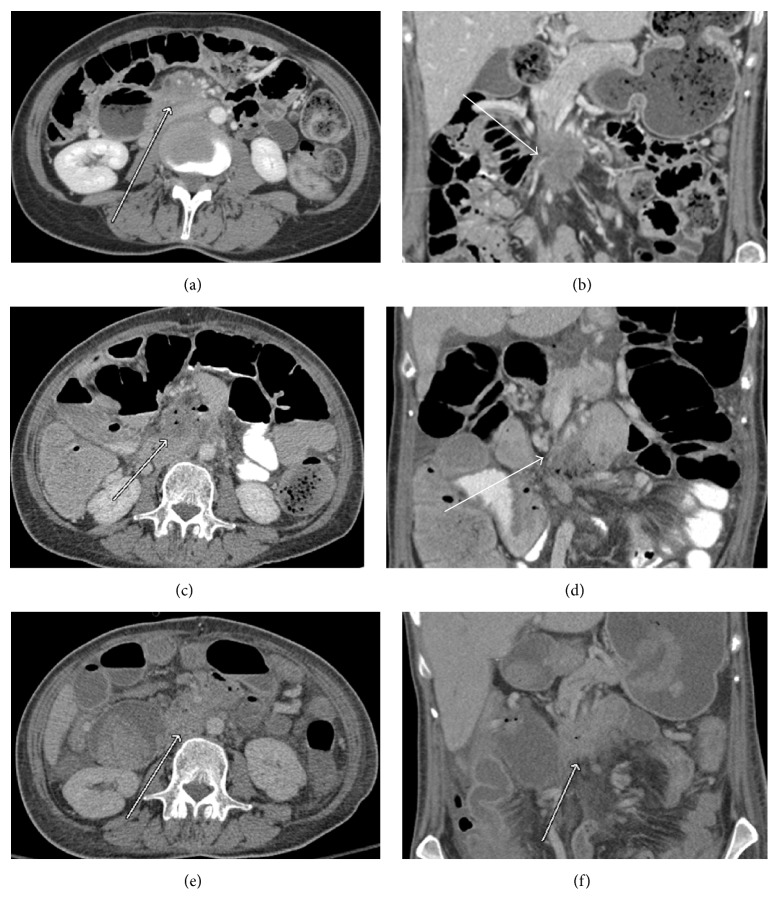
(a) and (b) Preoperative CT scans in axial and coronal planes showing soft tissue mass (white arrow) in the pancreatic uncinate process extending into the small bowel mesentery with vascular encasement. (c) and (d) Immediate postoperative CT scans demonstrating a slight fluid collection in postablative zone (white arrow) with ill-defined margins and internal gas compatible with liquefaction from ablation procedure. (e) and (f) Two-month follow-up scans demonstrating a smaller ablation zone (white arrow) with increased enhancement and less gas suggesting no recurrent disease.
